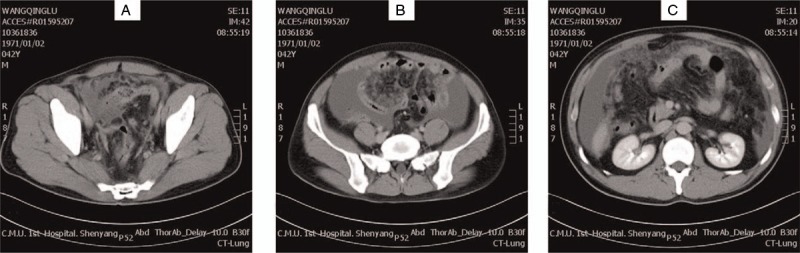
The preoperative computed tomography diagnosis of patient. (A) The intestine wall of the lesion had thickened at the recto-sigmoid junction. (B) Enhanced enteric cavity remaindered incomplete obstruction of intestine. (C) The density of intestinal mesentery and omentum increased obviously. There were amount of ascites and transferred nodes in abdominal cavity.
